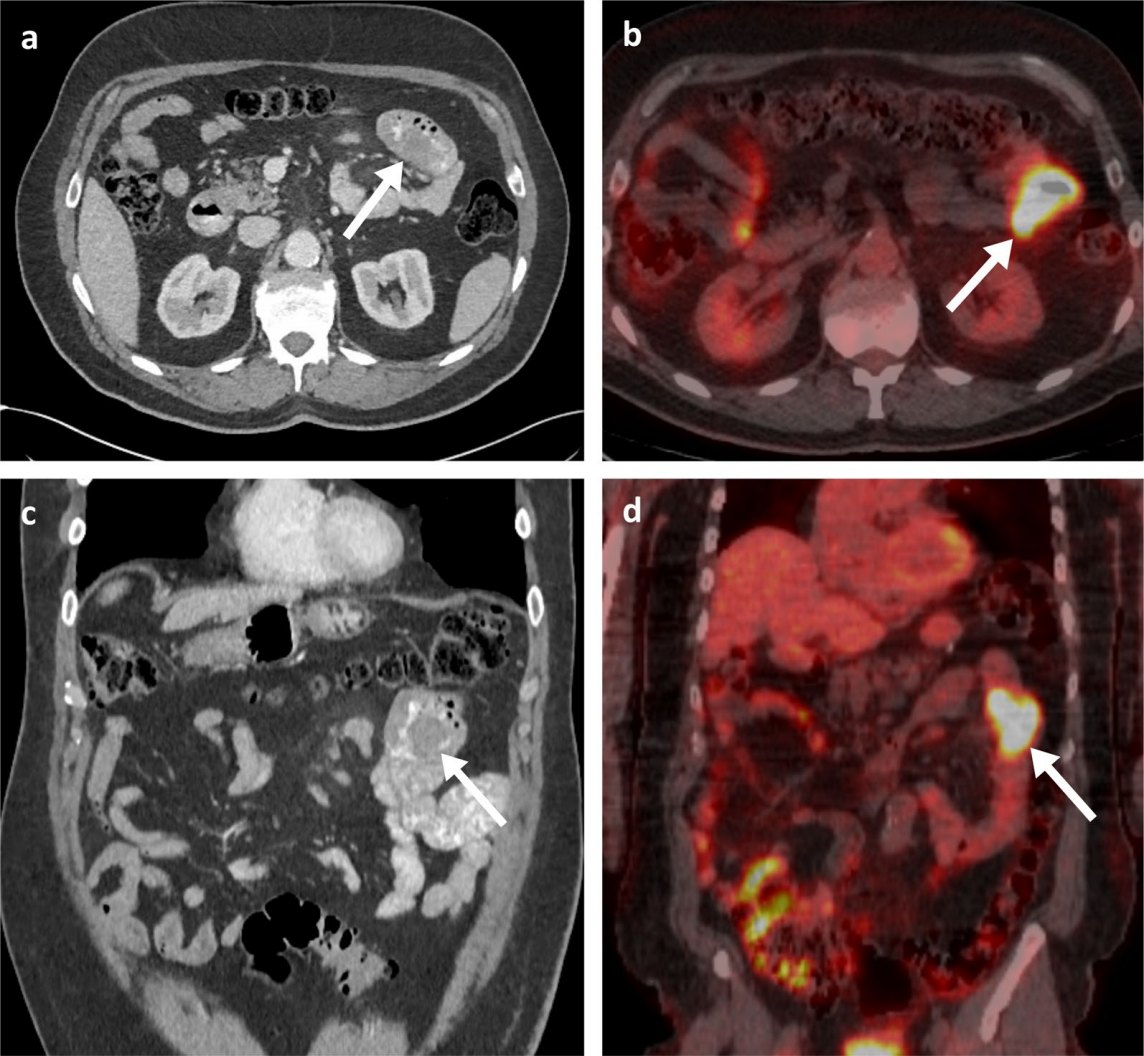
Oral and intravenous portal phase contrast-enhanced CT axial (a) and coronal (c) of a 70-year-old male patient with an eccentric mural melanoma metastasis within the proximal jejunum (arrows). PET-CT axial (b) and coronal (d) demonstrating FDG avidity with an SUVmax of 21.7 (arrows)
